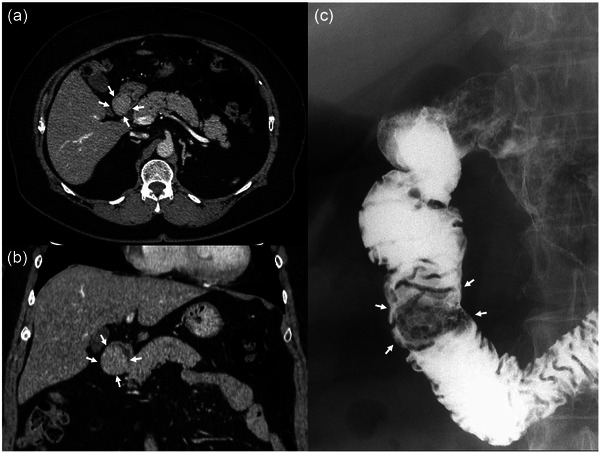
Computed tomography and duodenography findings. Enhanced computed tomography image shows a homogeneous, well‐circumscribed mass with a diameter of 30 mm in the second portion of the duodenum (a: axial image; b: coronal image; arrows). (c) A duodenography reveals a 30‐mm well‐defined mass in the second portion of the duodenum (arrows).
